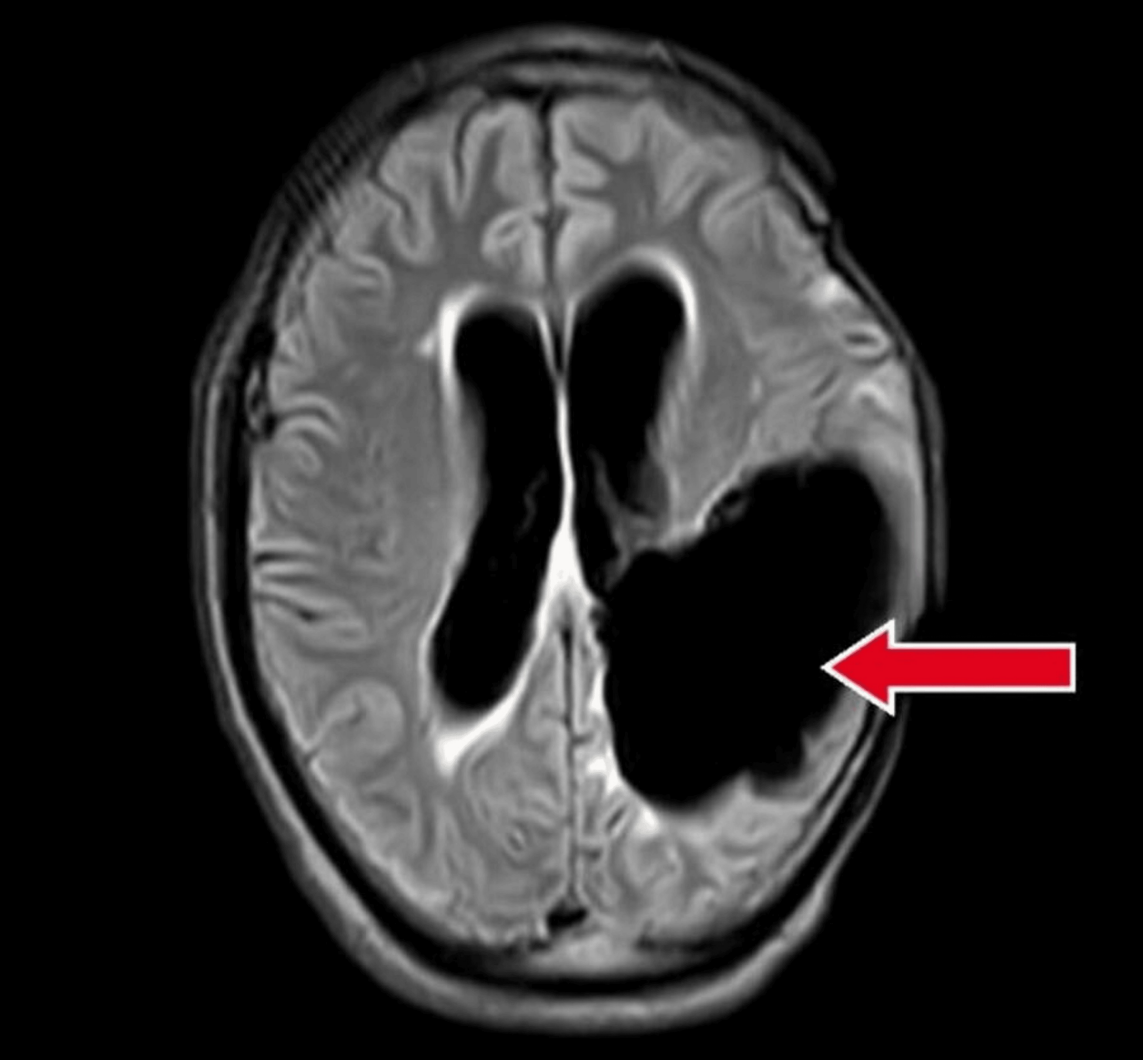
FLAIR magnetic resonance imaging showing lesion in the parietal and occipital lobes with red arrow. FLAIR: fluid-attenuated inversion recovery
